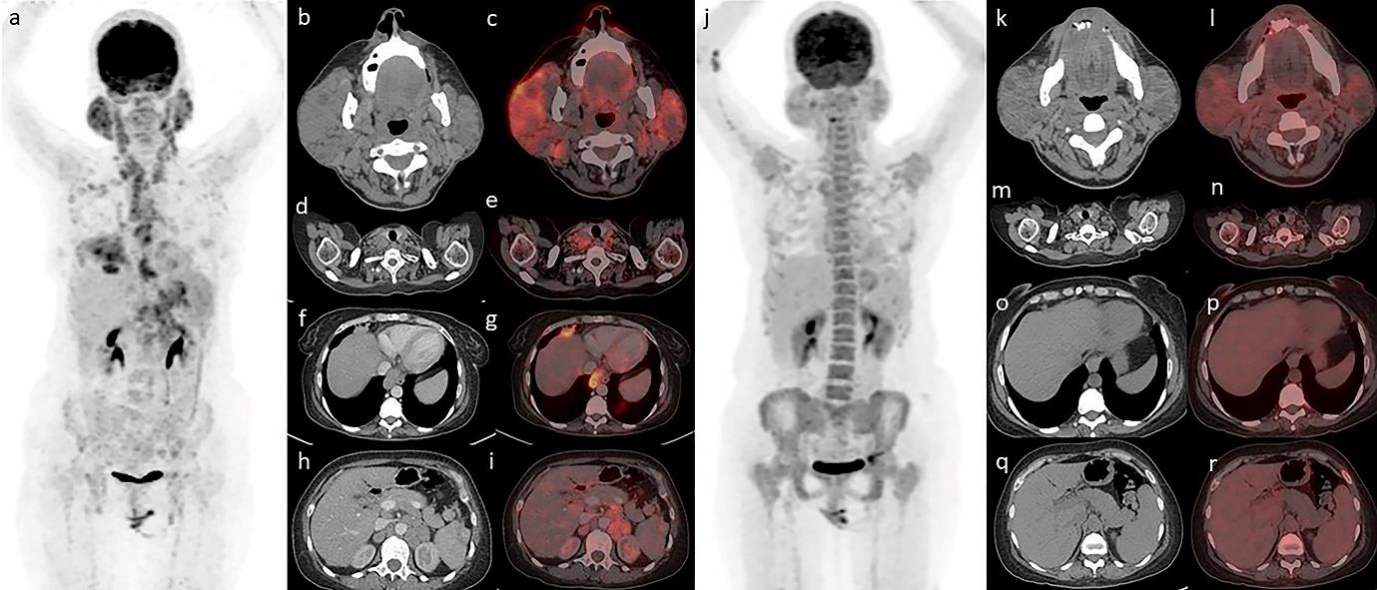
FDG PET/CT showed metabolically active enlarged bilateral parotid (b,c) and lacrimal glands with cervical, supraclavicular (d,e), mediastinal, anterior diaphragmatic (f,g), and abdomino-pelvic lymph nodes (h,i) along with hepatosplenomegaly. Compared to previous PET/CT scan (j, k, r) there was an increase in size and uptake of the bilateral parotid glands along with the new lesions
